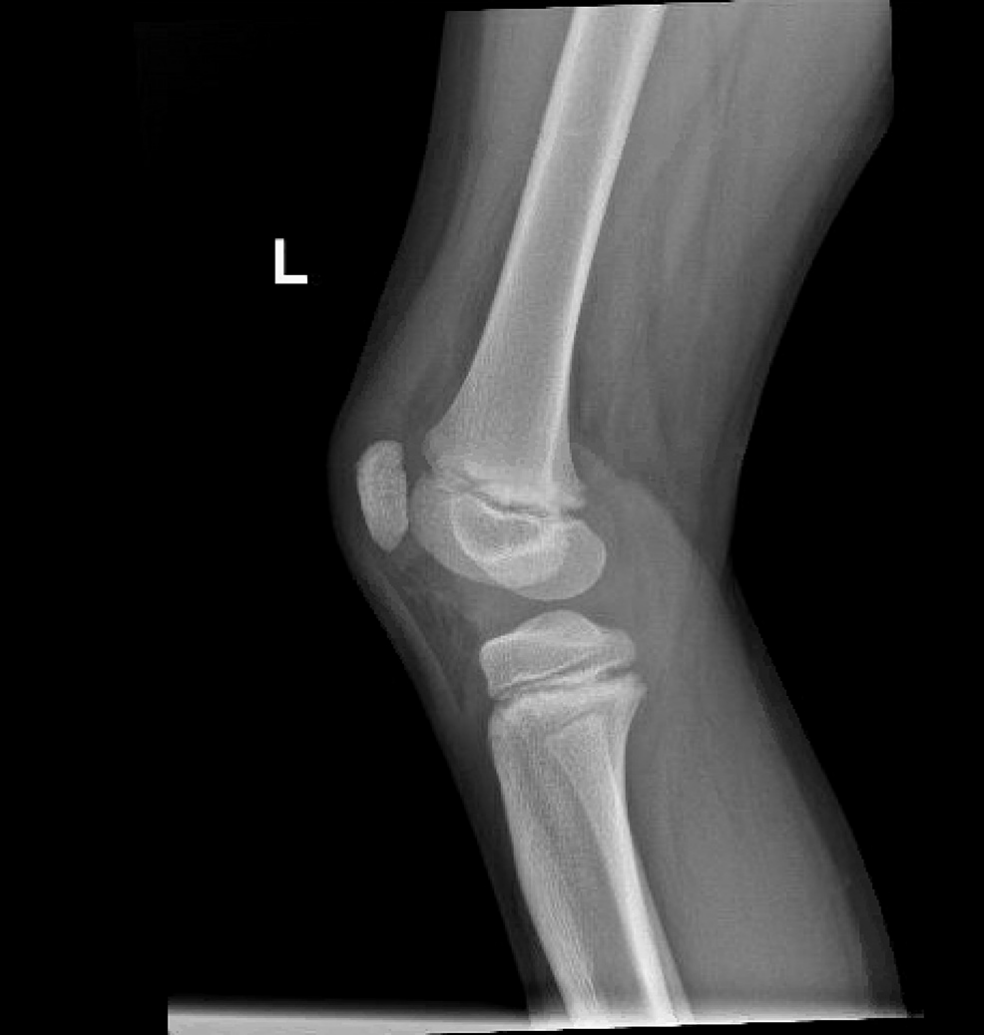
Lateral x-ray view of the left knee.
